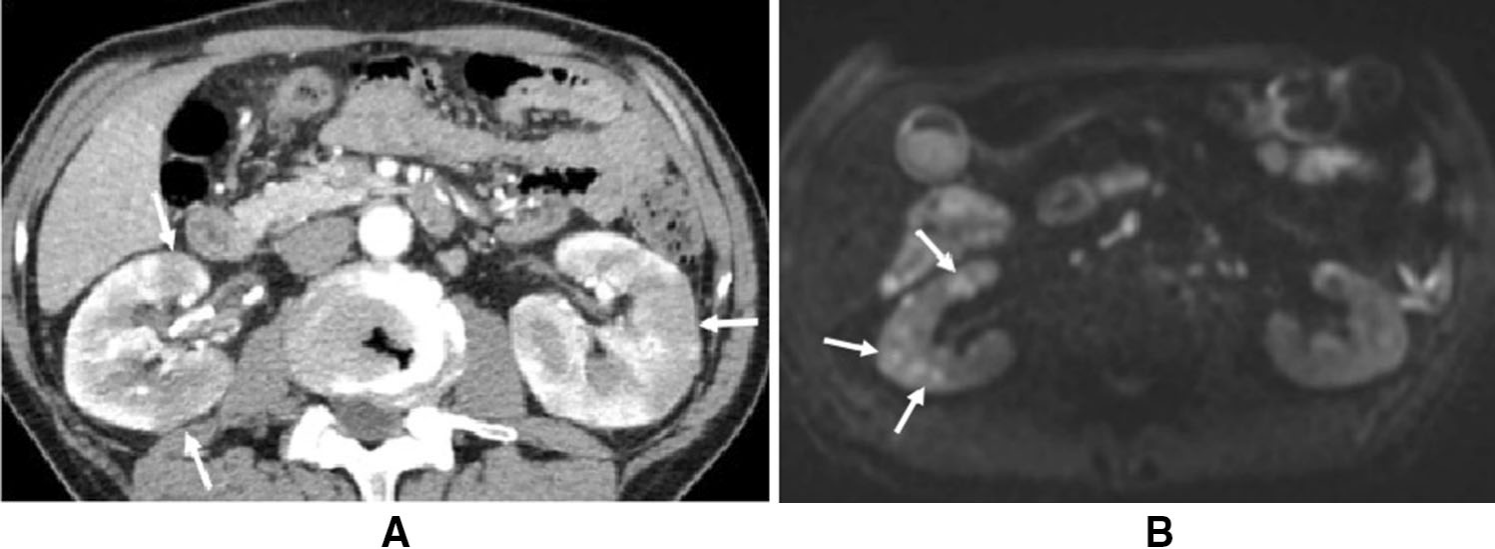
CT/MRI images of AIP-related kidney disease (AIP-KD). A Dynamic contrast-enhanced CT (arterial phase) shows multiple poorly enhanced nodules in both renal cortexes (arrows). B Diffusion-weighted MRI reveals multiple decreased diffusion areas in the right kidney (arrows)
